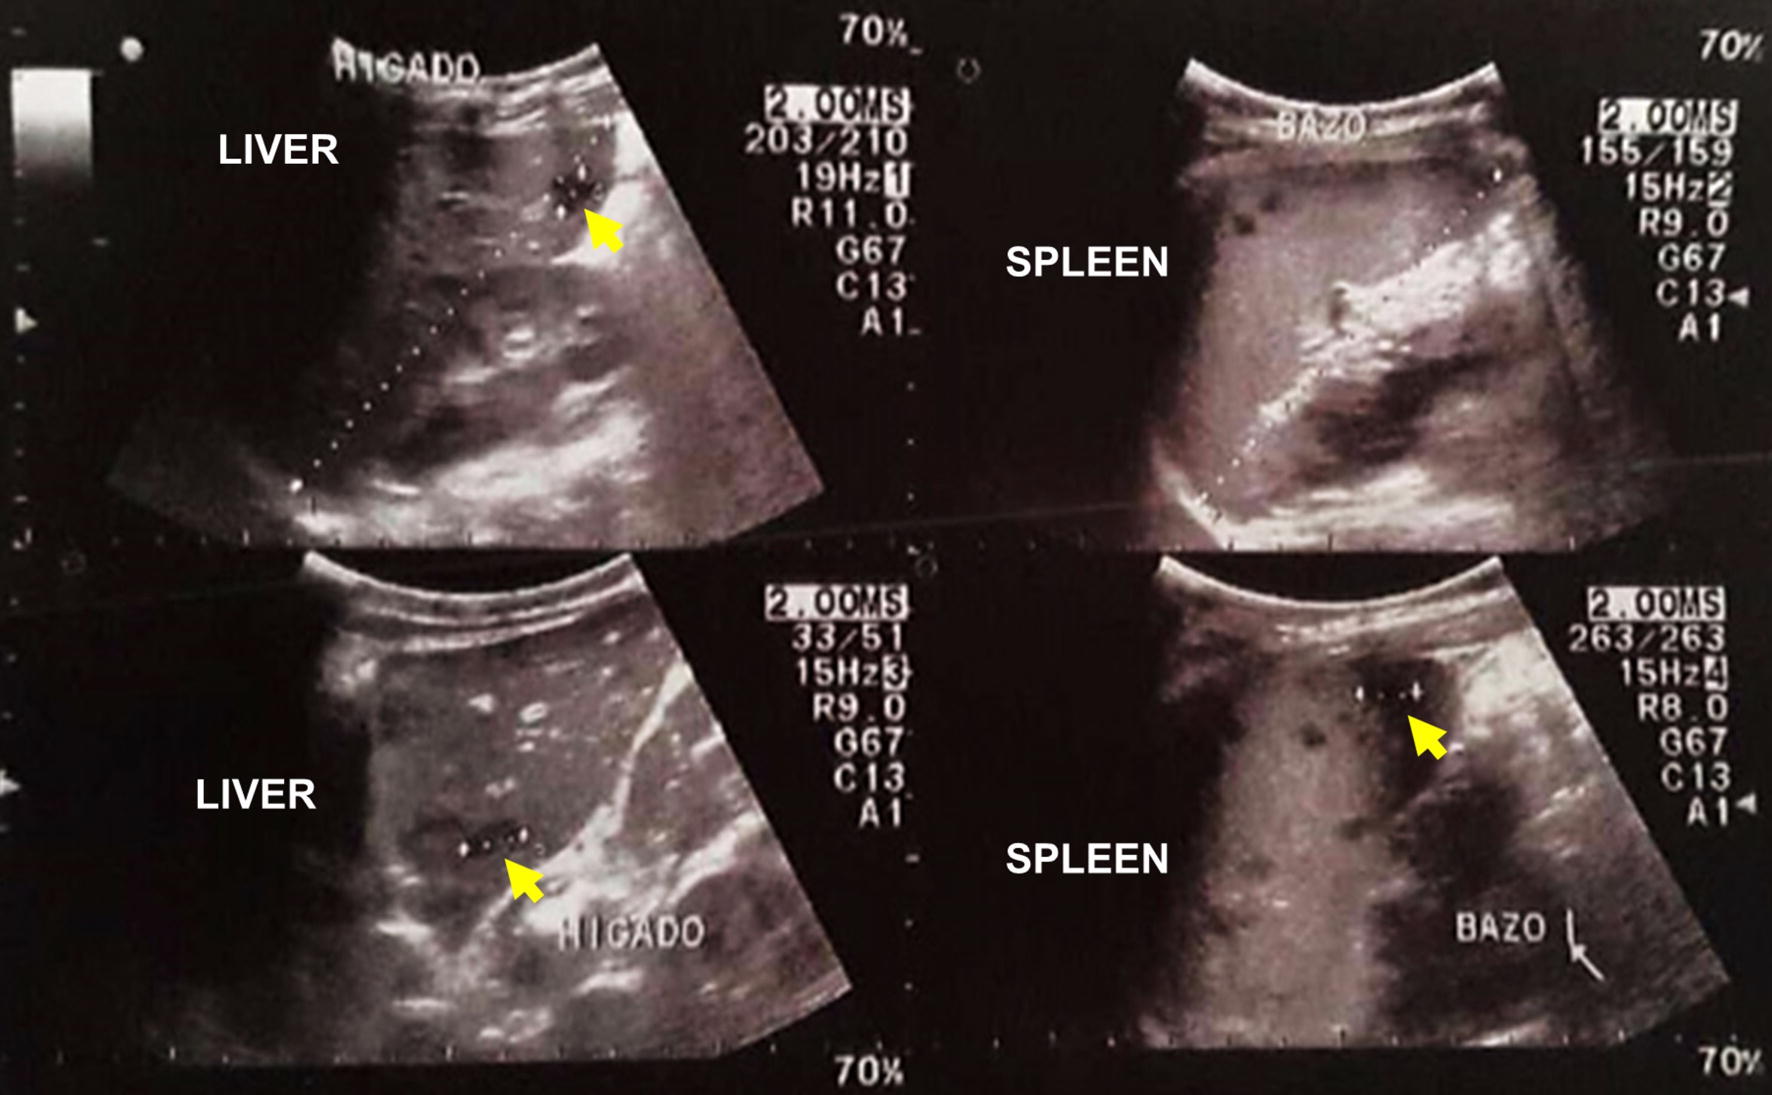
Abdominal ultrasound showing multiple hypoechoic areas with poorly defined edges of up to 11 mm in the liver and in the spleen (yellow arrows indicate the lesions)
